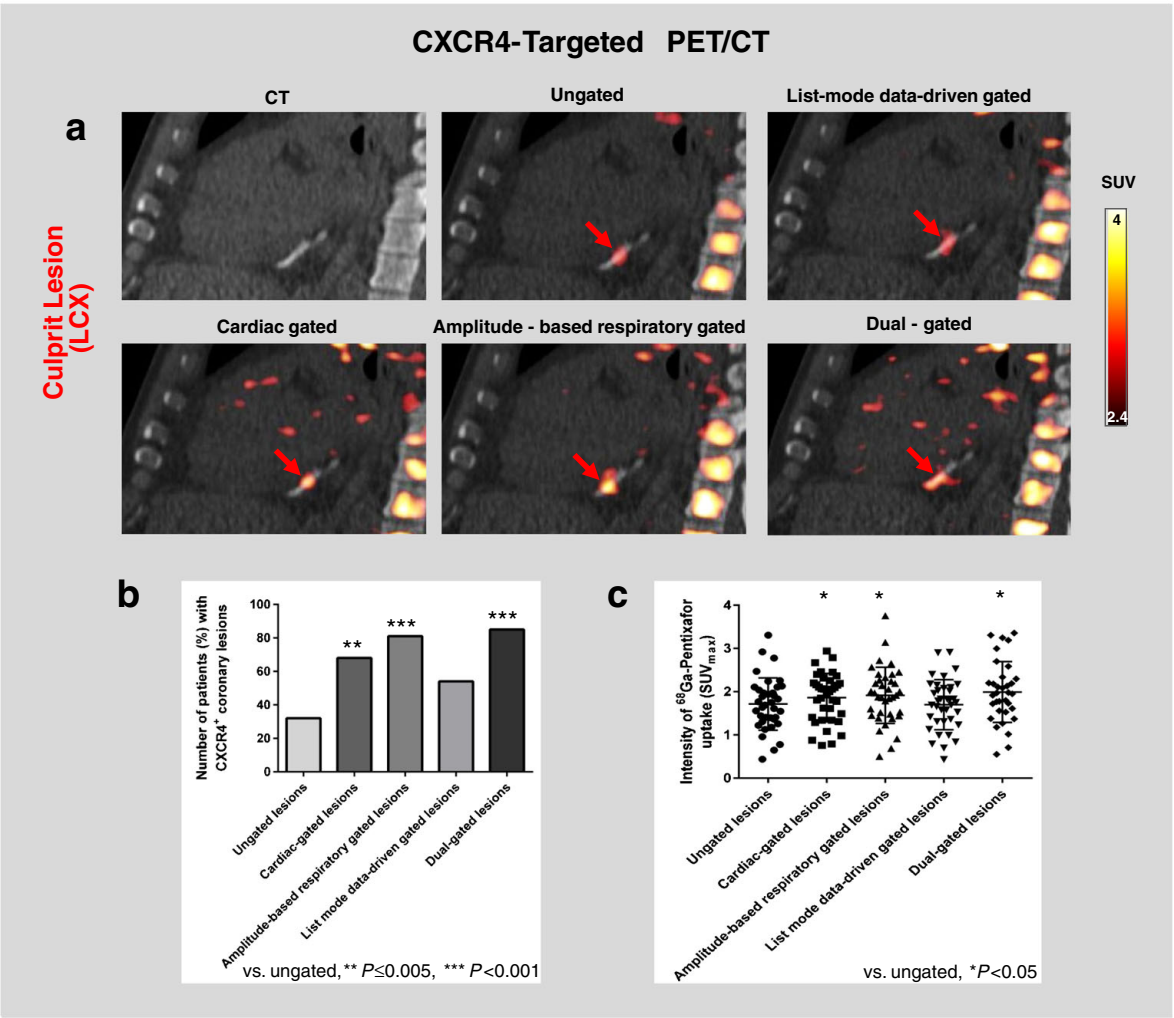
Effect of motion correction on [^68^Ga]pentixafor uptake and detectability in culprit coronary lesions. a [^68^Ga]Pentixafor PET/CT images of a culprit LCX lesion. Focal CXCR4 signal (*red*) is more clearly depicted after cardiac, respiratory motion or dual cardiac/respiratory motion correction (*lower row*) compared to ungated images (*upper row*, *middle*). b Detection rates for CXCR4^+^ culprit coronary lesions are higher in gated images (*P* ≤ 0.005). c Signal intensity of CXCR4^+^ culprit coronary lesions is higher in gated images (*P* < 0.05). Background subtraction was performed using an individually adjusted threshold in this particular subject for clearer visualization of tracer uptake
